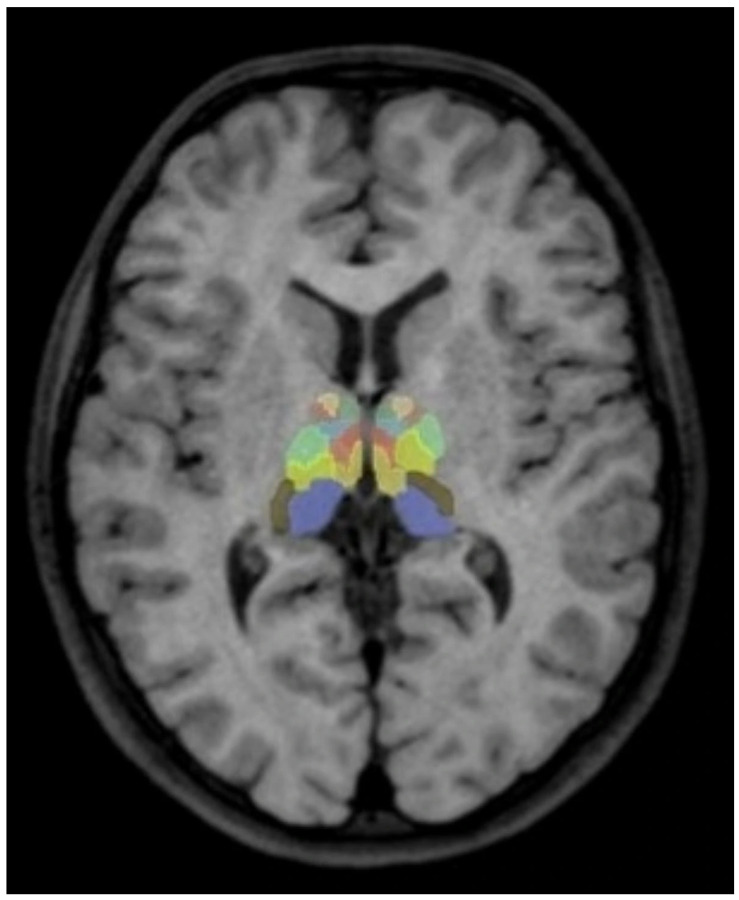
Representative axial three-dimensional (3D) T1-weighted magnetic resonance image demonstrating automated color-coded segmentation of bilateral thalamic nuclei using the FreeSurfer thalamic nuclei module. Distinct colors correspond to anatomically defined thalamic nuclei included in the volumetric analysis: anteroventral nucleus (AVN, orange), mediodorsal nucleus (MN, salmon-red), ventrolateral anterior nucleus (VLAN, yellow), ventral anterior nucleus (VAN, light green), ventral posterolateral nucleus (VPLN, dark green), lateral geniculate nucleus (LGN, cyan), medial geniculate nucleus (MGN, light blue), intralaminar nuclei (ISN, purple), and pulvinar nucleus (PN, dark blue).
